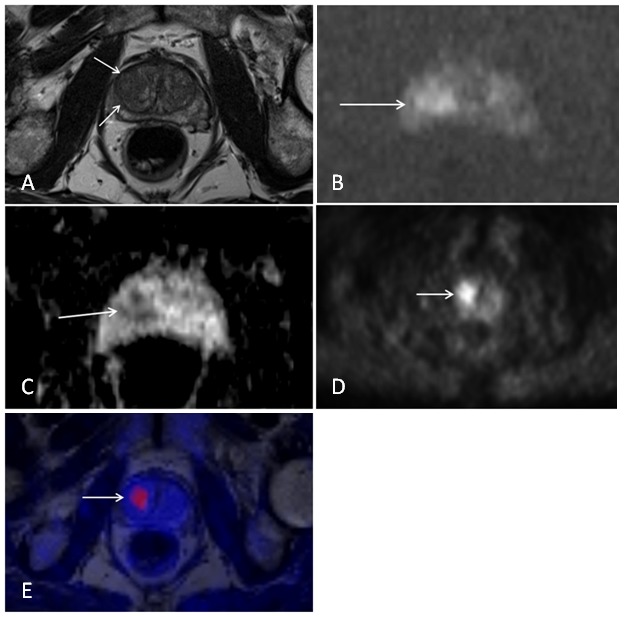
Patient with a biopsy proven prostate cancer of the right transitional zone (Gleason score 3+4 = 7). Images from simultaneous [^18^F] choline PET/MRI. A) T2-weighted image showing an ill-defined hypo-intense lesion of the right transitional zone. B) Diffusion-weighted image at a b-value of 1000 displaying a hyper-intense signal within the lesion indicating restricted water diffusion. C) ADC map with a corresponding hypo-intensity of the lesion. D) PET image showing a focal uptake of (18F) choline of the lesion. E) Fused MRI/PET image.
